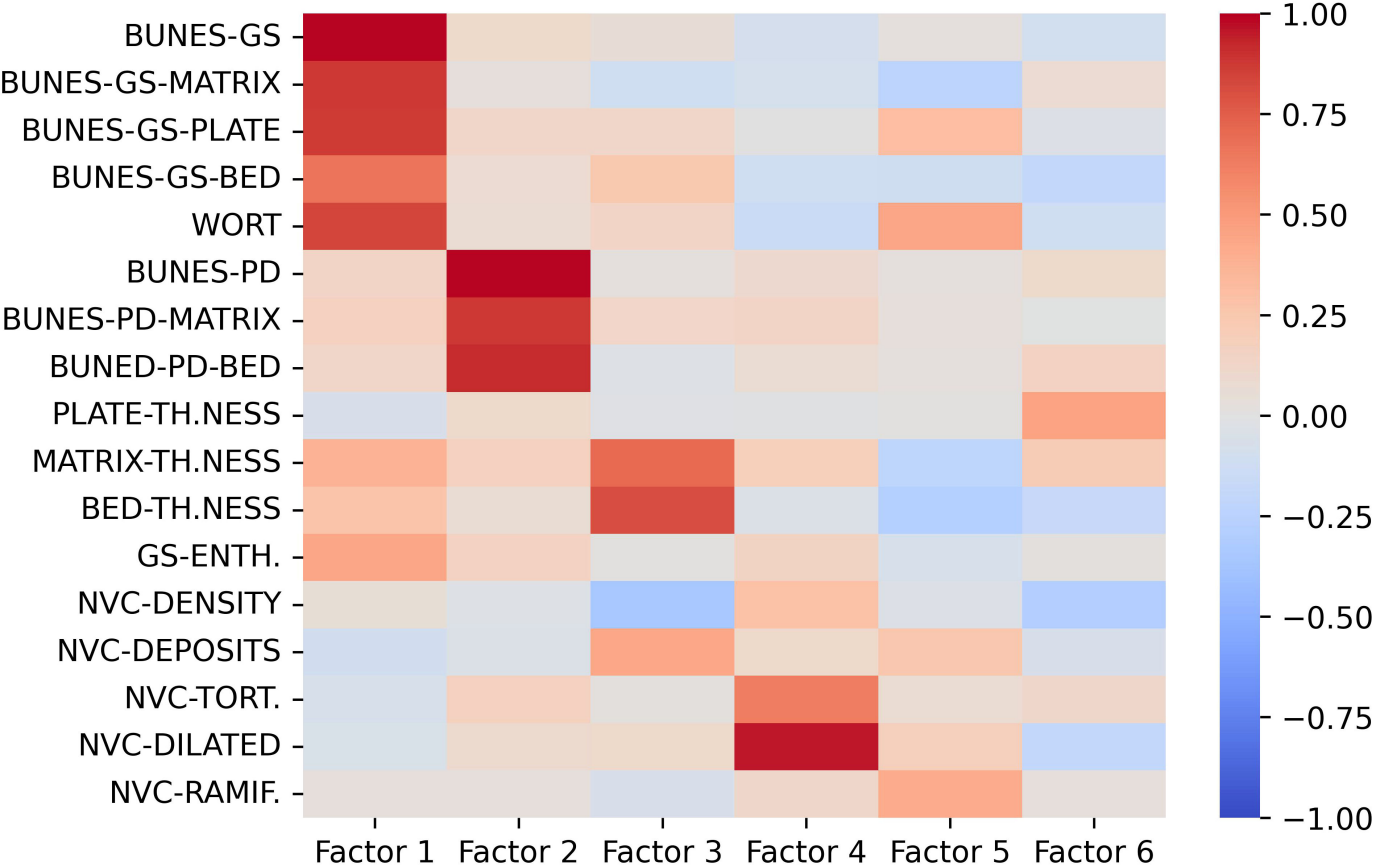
Loading values of US and NVC variables on the first 6 factors. Gray-scale US parameters mostly load on Factor 1, US PD features mostly load on Factor 2, Nail-enthesis quantitative measures mostly load on Factor 3, NVC-detected ectasia and tortuosities mostly load on Factor 4. The remaining variables do not seem to provide major contribution to the clustering model. BUNES, Brown University Nail Enthesis Scale; GS, grayscale; PD, Power-Doppler.
